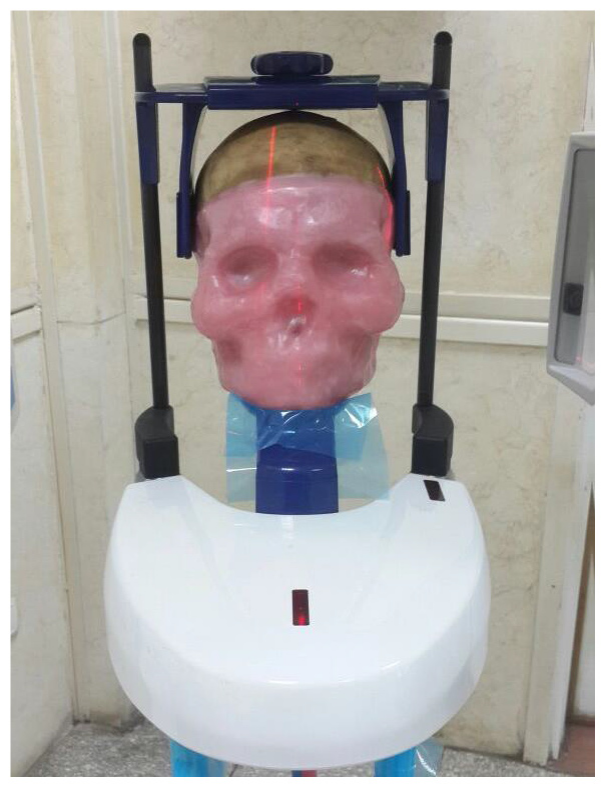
A photograph showing the skull centralized within the CBCT machine in the proper position.
